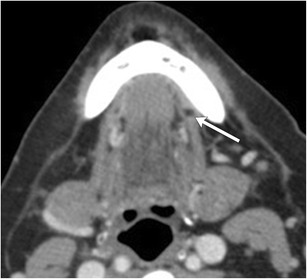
Mylohyoid slit (boutonniere). Axial contrast-enhanced CT image shows a linear, fat-containing defect within the mylohyoid muscle at the anterior aspect (*arrow*)
